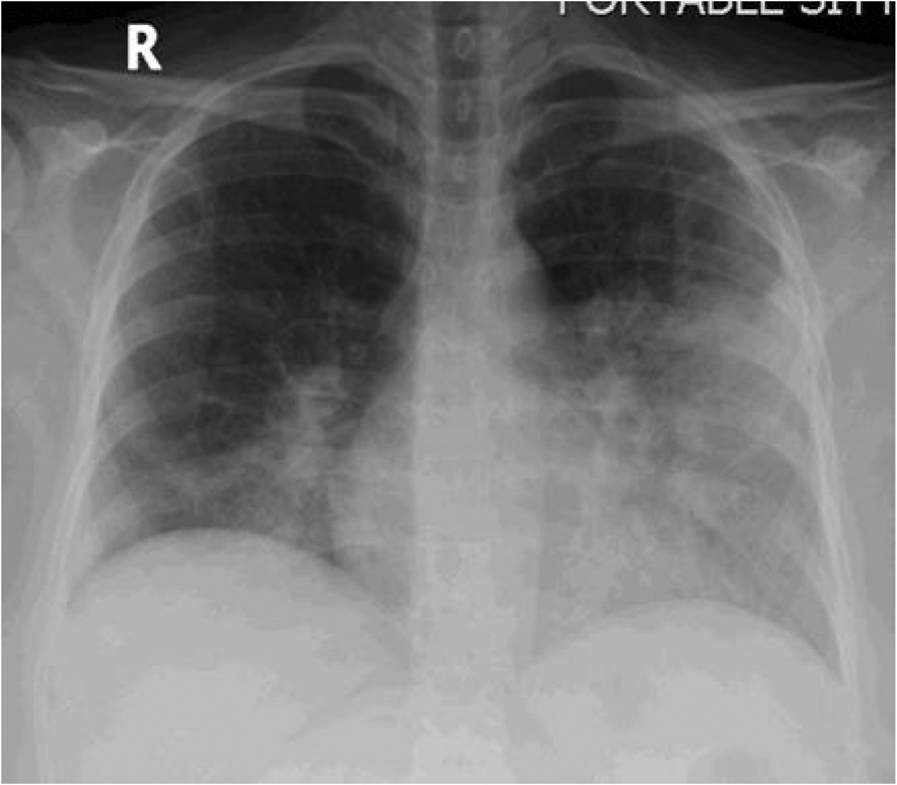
Chest Radiography (postero-anterior view) showing bilateral infiltration
